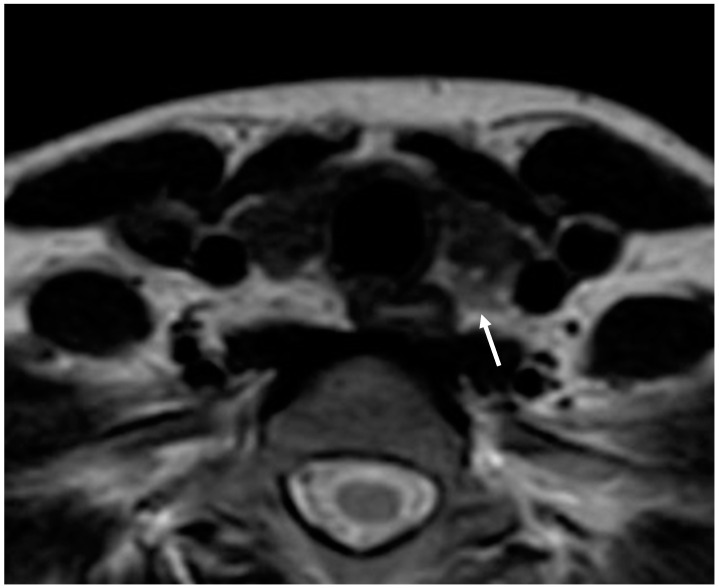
Parathyroid adenoma. Axial T2-weighted image shows a small iso-hyperintense mass behind the left thyroid lobe (arrow).
